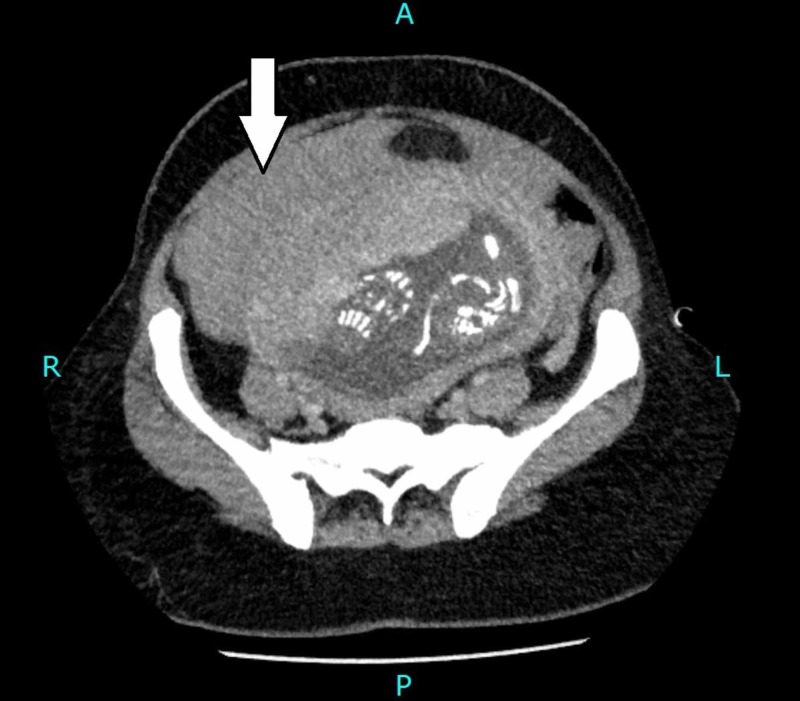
Computed tomography image (axial section). High-density free fluid with Hounsfield units of 80, adjacent to the uterus, suggestive of hemoperitoneum (arrow).
